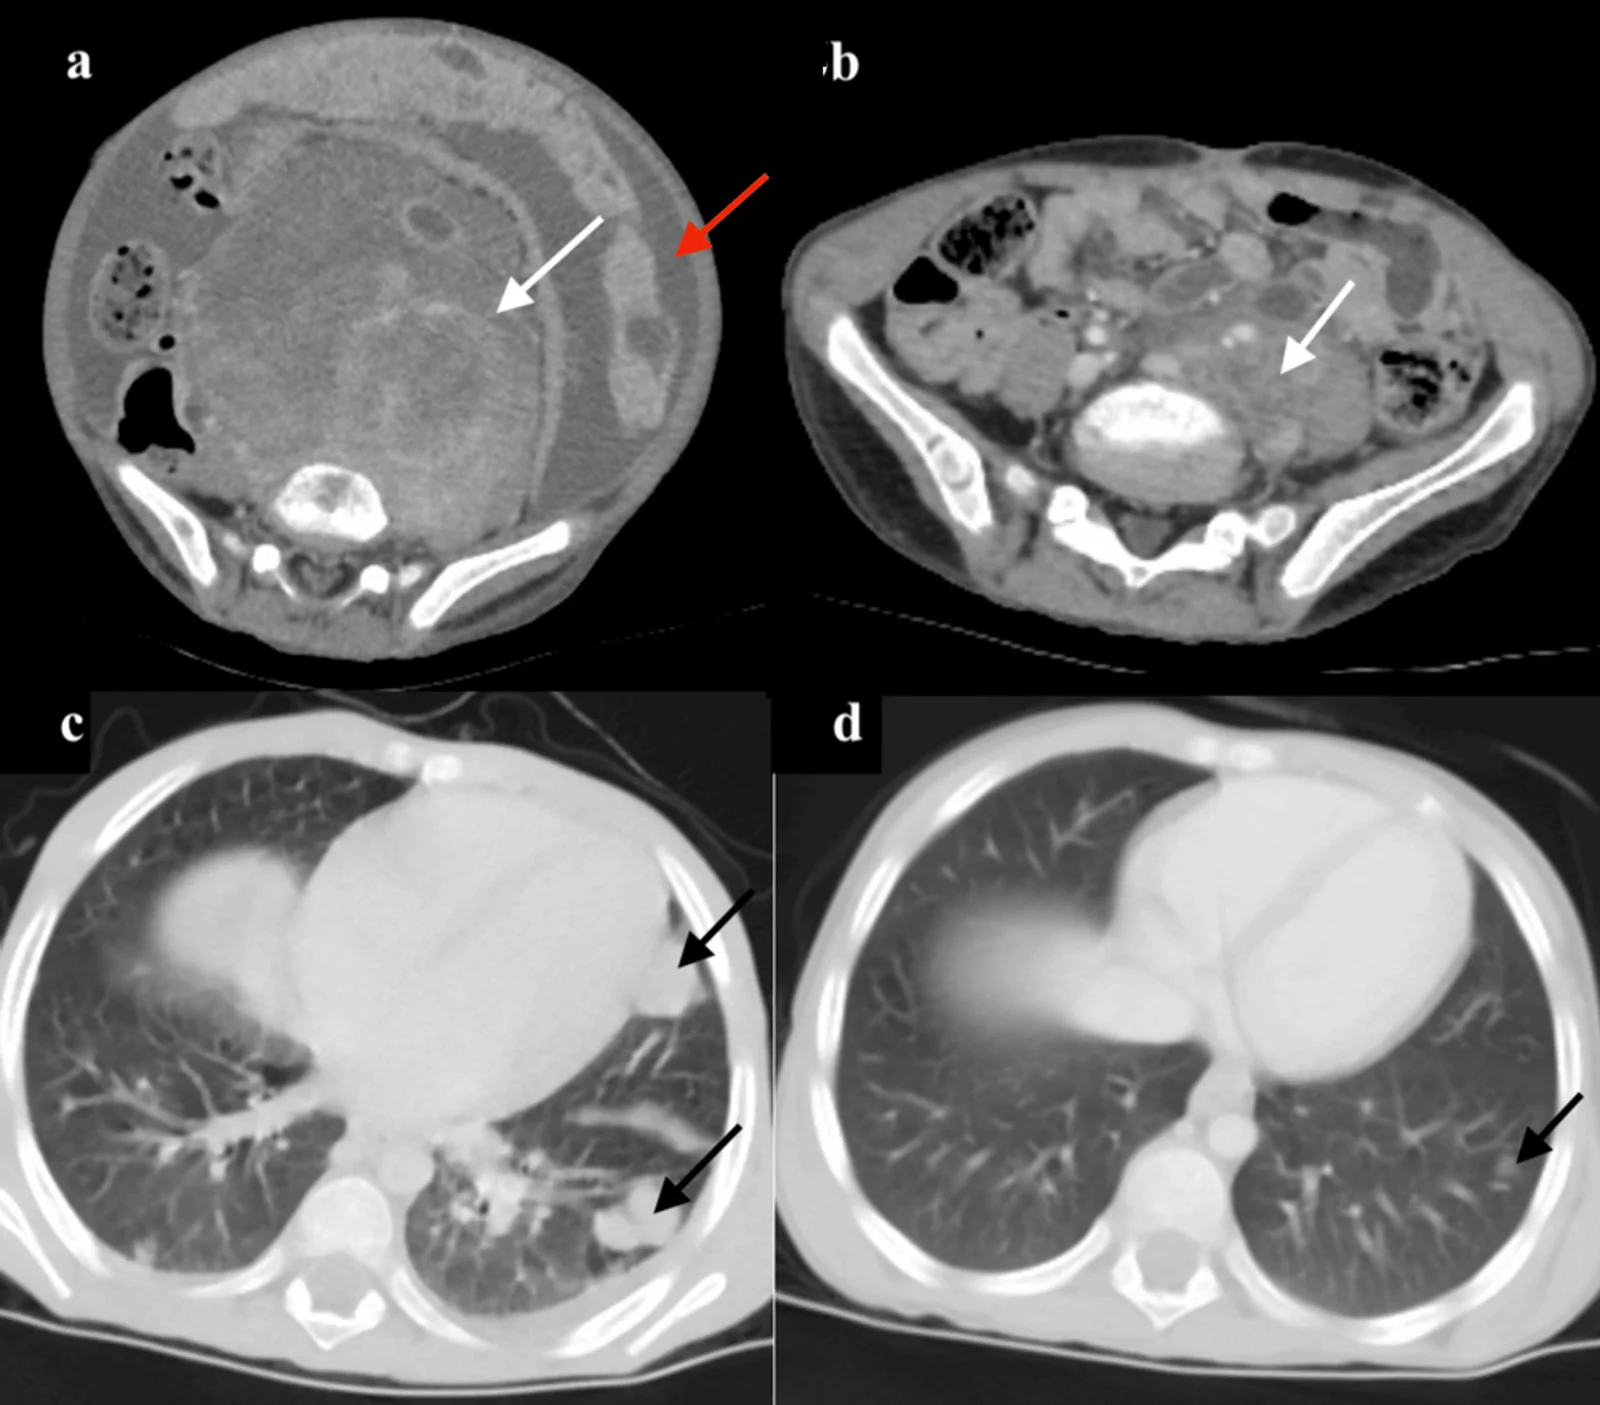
Comparative CT before (a, c) and after (b, d) induction chemotherapy. (a, b) Abdominopelvic CT showing marked regression of the retroperitoneal mass (white arrow) and resolution of the peritoneal effusion (red arrow). (c, d) Chest CT showing near-complete resolution of the pulmonary nodules (black arrows).
